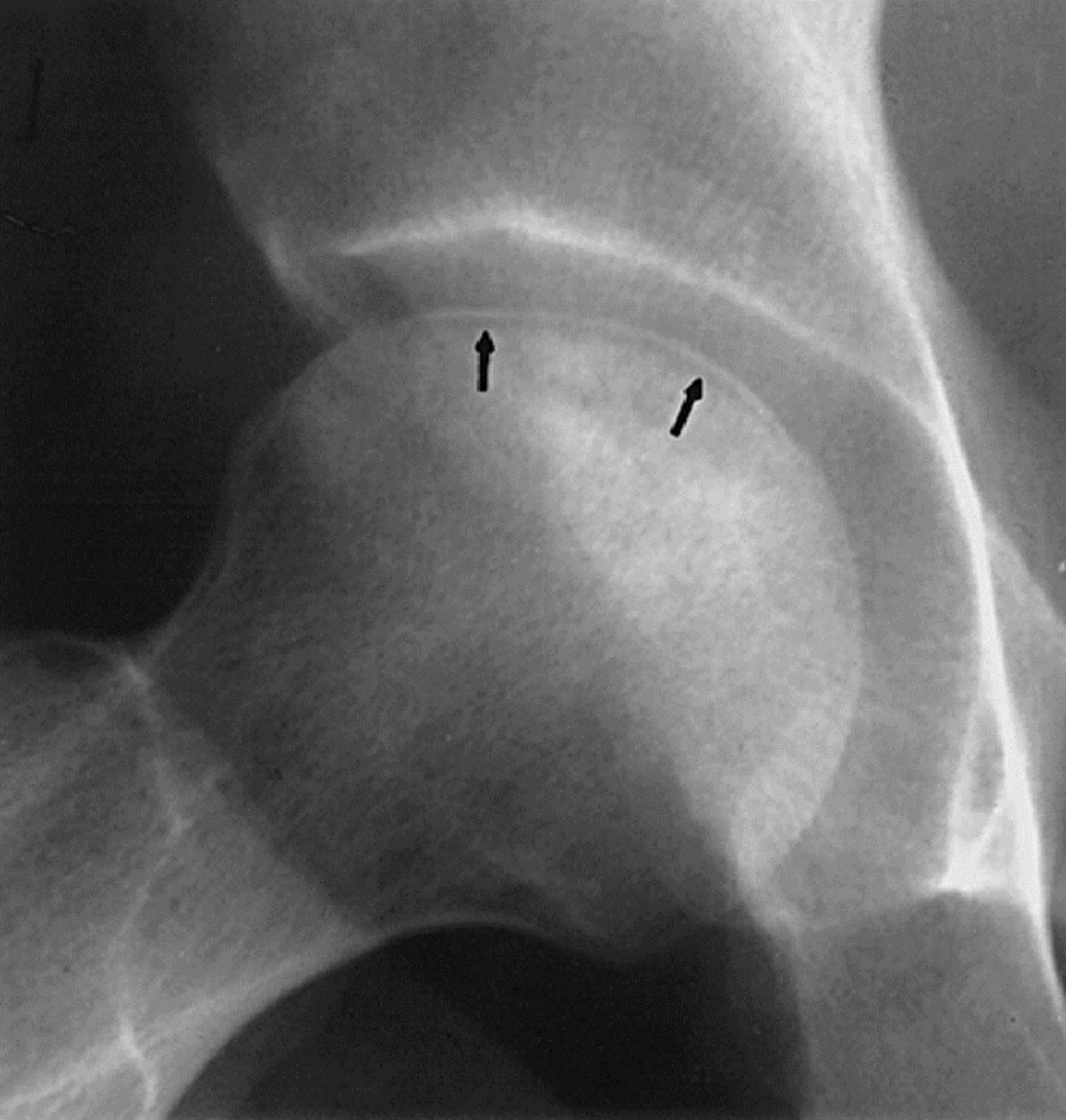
X-ray hip Crescent sign. Arrows showing the hypointense crescent. (Courtesy http://onradiology.blogspot.com)
